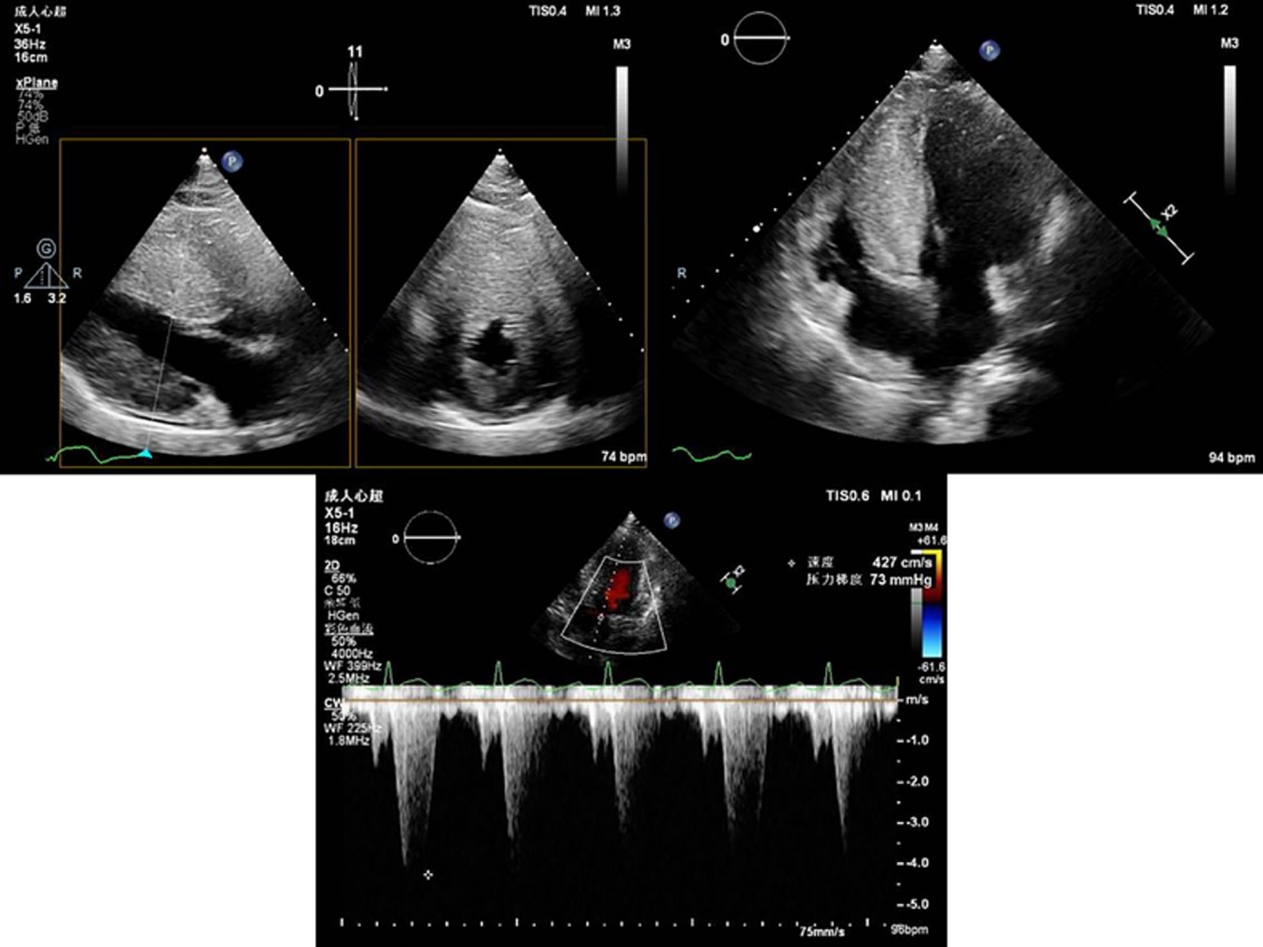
Echocardiogram. The echocardiogram demonstrated hypertrophy involving the interventricular septum, left ventricular anterior wall and apex, and severe LVOT obstruction and RVOT obstruction. The peak gradient across the LVOT was 73 mmHg, and the RVOT was 23 mmHg at rest
